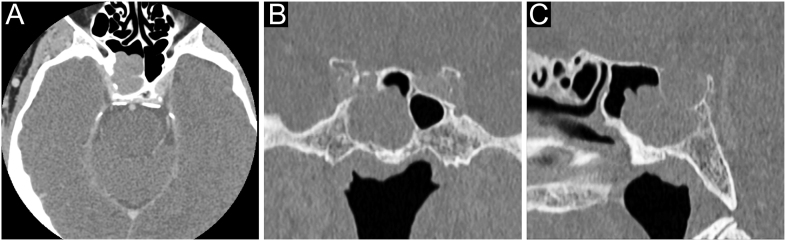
A TSH-secreting PitNET in a 74-year-old man. This patient came to our observation with a previous history of goitre and hyperthyroidism treated with anti-thyroid medications followed by total thyroidectomy. He had received increasing doses of levothyroxine for persistently elevated TSH and had a pacemaker implanted for the management of arrhythmias and atrial fibrillation. Due to iatrogenic hyperthyroidism, replacement therapy was reduced from 175 to 75 μg/dL, leading to a further increase in plasma TSH (15 µIU/mL) when peripheral euthyroidism was restored. Basal PRL and GH were normal (17 and 1.3 ng/mL, respectively) with low IGF1 levels (45 ng/mL). He denied headaches or visual disturbances, but a contrast-enhanced head CT revealed a sellar mass (27 × 21 mm) with parasellar and infrasellar invasion. Transsphenoidal surgery was then performed, and pathological findings concluded for a mixed TSH/PRL immunoreactivity with no mitoses and Ki-67 expression (3%). Based on post-operative residual biochemical and radiological disease, the patient was successfully treated with first-generation somatostatin receptor ligands, with a good tolerance and efficacy over a 4-year follow-up. (A) Coronal contrast-enhanced T1-weighted scan, (B) coronal T2-weighted scan and (C) sagittal contrast-enhanced T1-weighted scan.
